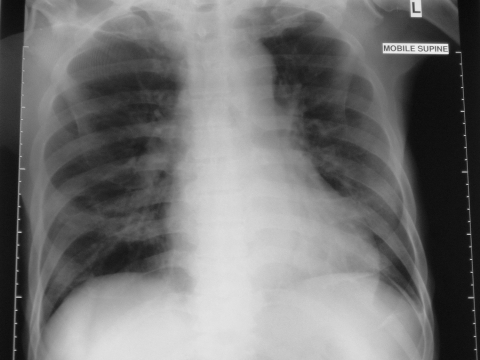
Radiograph on day 11 of hospital stay (day 14 after contact with a SARS patient).
